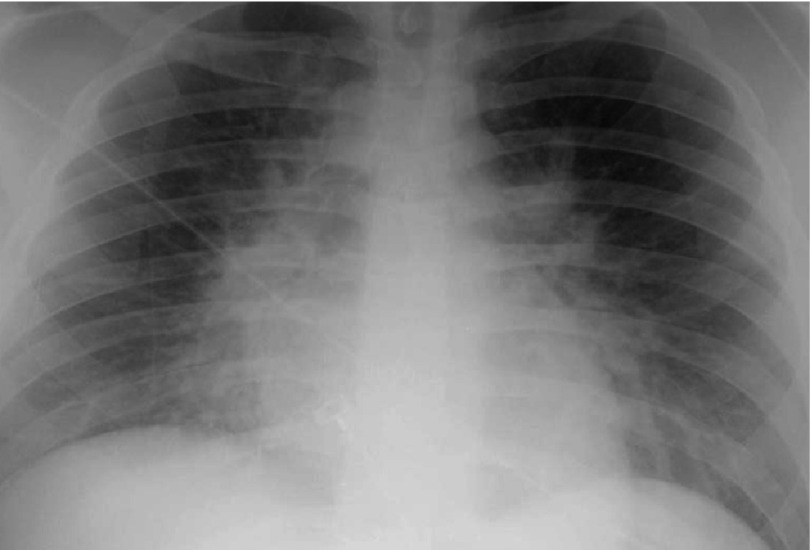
Chest x-ray showing moderate to severe pulmonary edema with borderline heart size.
